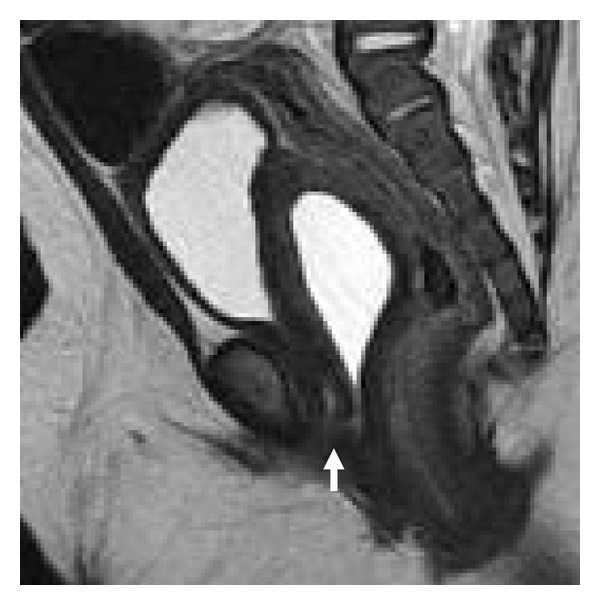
Sagittal T2 TSE MR image revealing the communication between the prostatic utricle cyst and the urethra (white arrow).
